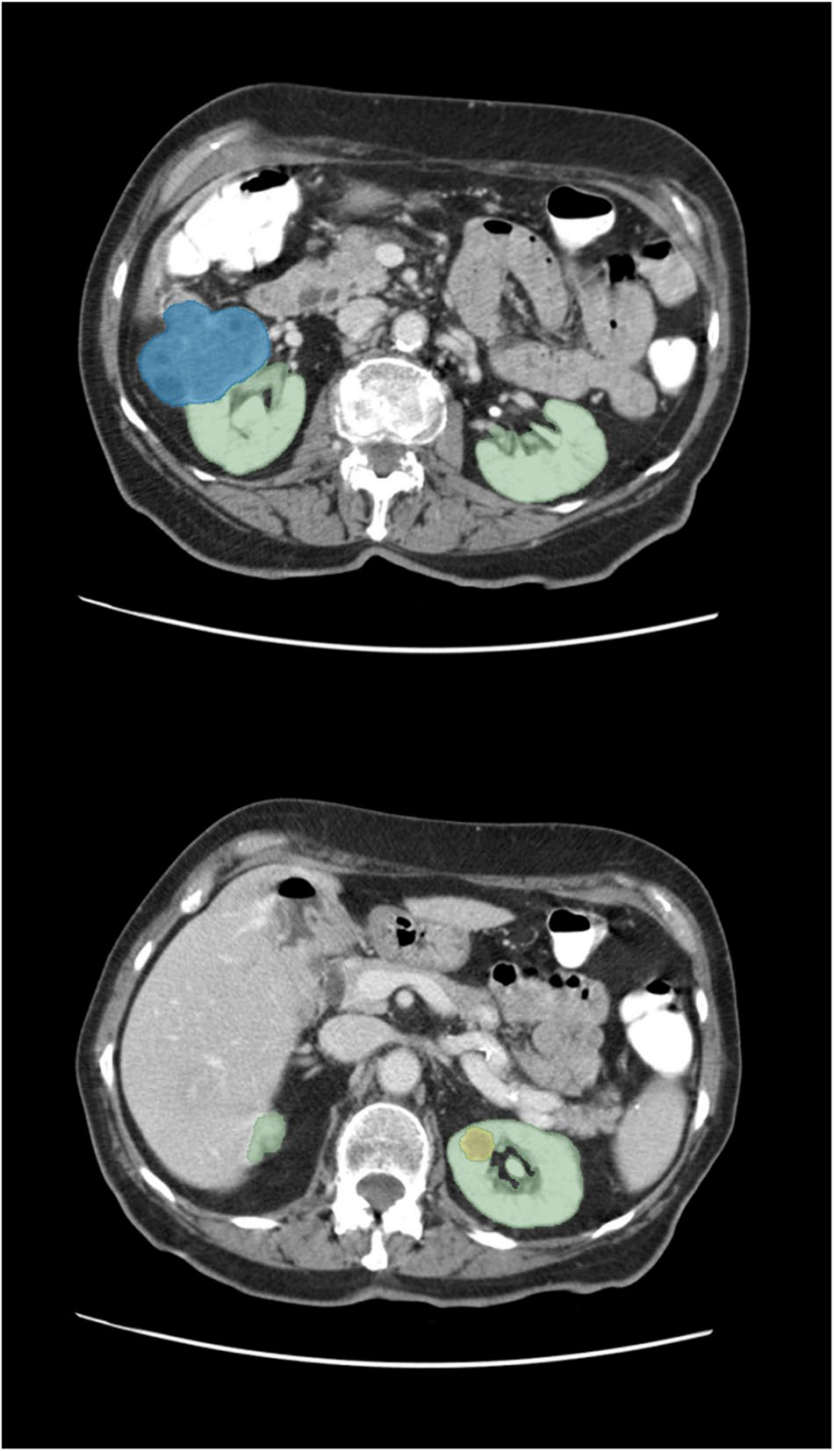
Automatic segmentation of kidney (green), tumor (blue), cyst (yellow) from CT scan of patient TCGA-CJ-4873.
